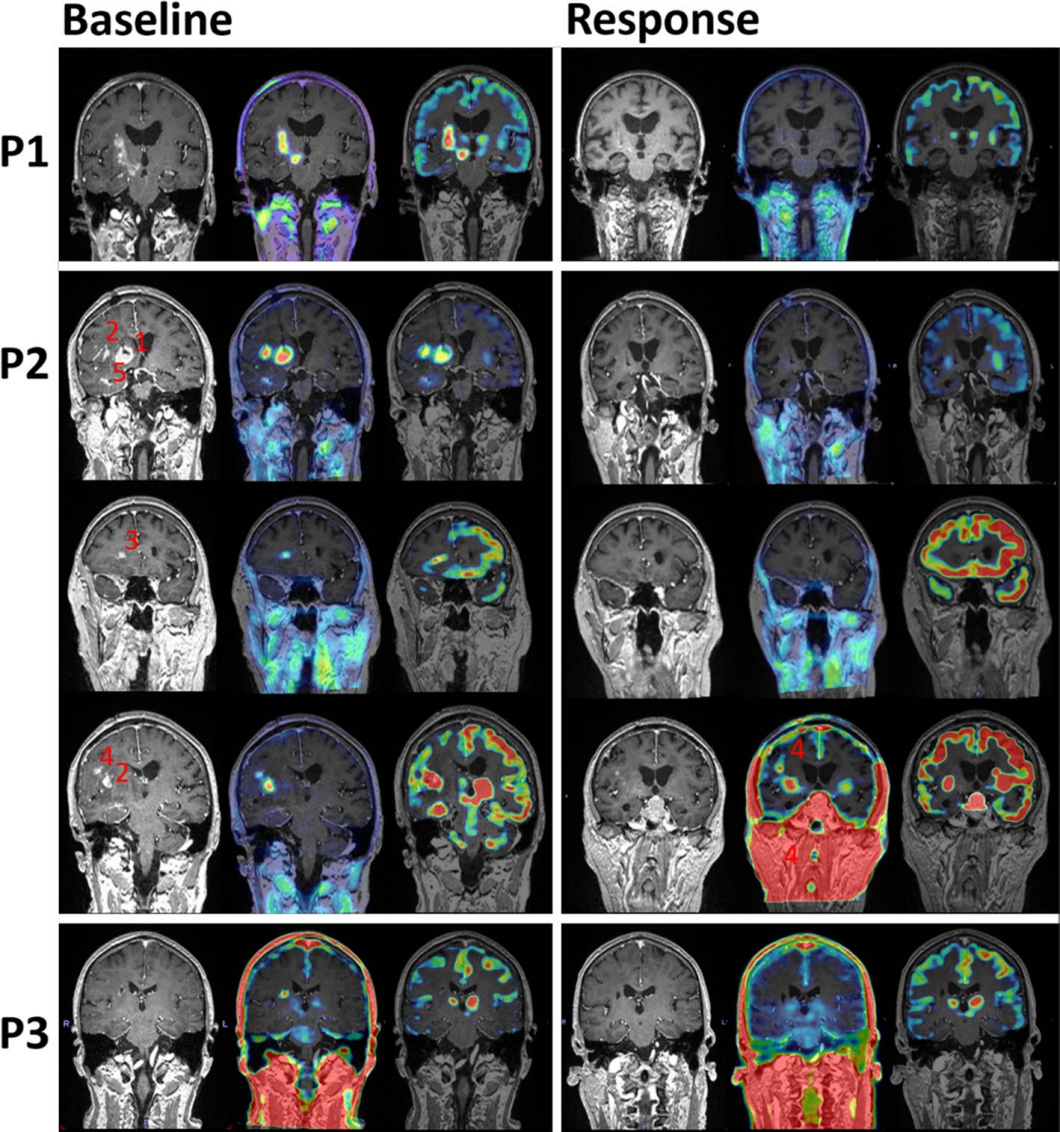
T1 Ce-MRI, fused ^18^F-FACBC/ce-MRI, and fused ^18^F-FDG/ce-MRI are shown from left to right. The left and right panel show baseline and response PET/MRI images, respectively. Patients 1–3 (P1–3) are distributed vertically. Scale for ce-MRI was 0–600. Scale for PET detected lesions was SUV_background_ − SUV_max._ Scale for PET undetected lesions was SUV_background_—SUV_max_ (baseline lesion uptake). For P3 ^18^F-FDG scans, the lesion was undetected in both scans and the scale used was SUV_background (mean)_ − SUV_background (max)_
